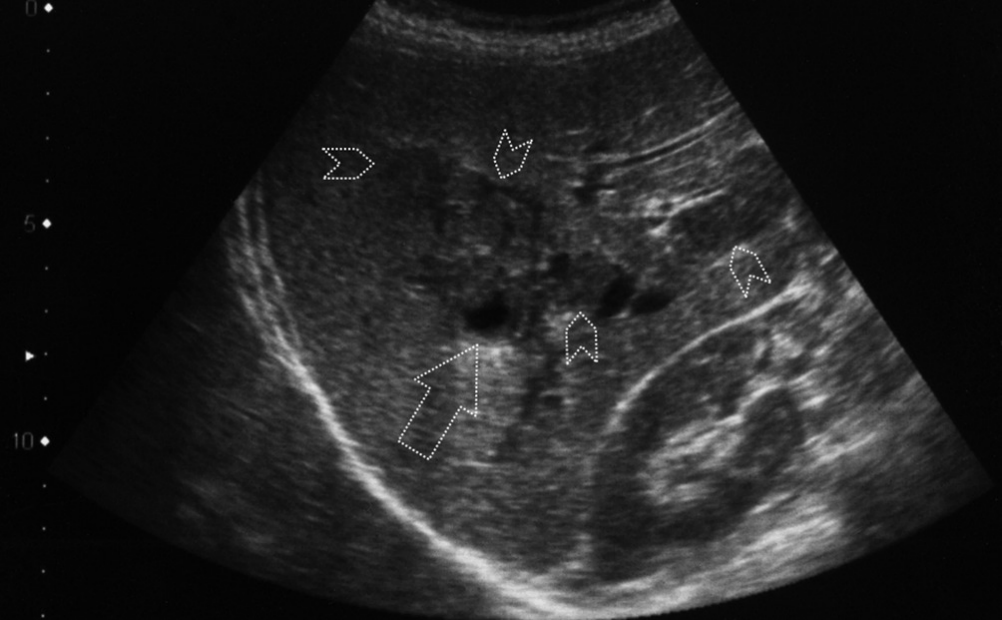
Ultrasound image of the liver shows multiple, ill-defined hypoechoic confluent nodular masses (chevron arrows). Note the central necrosis area in one of the larger-sized lesions (large arrow).
